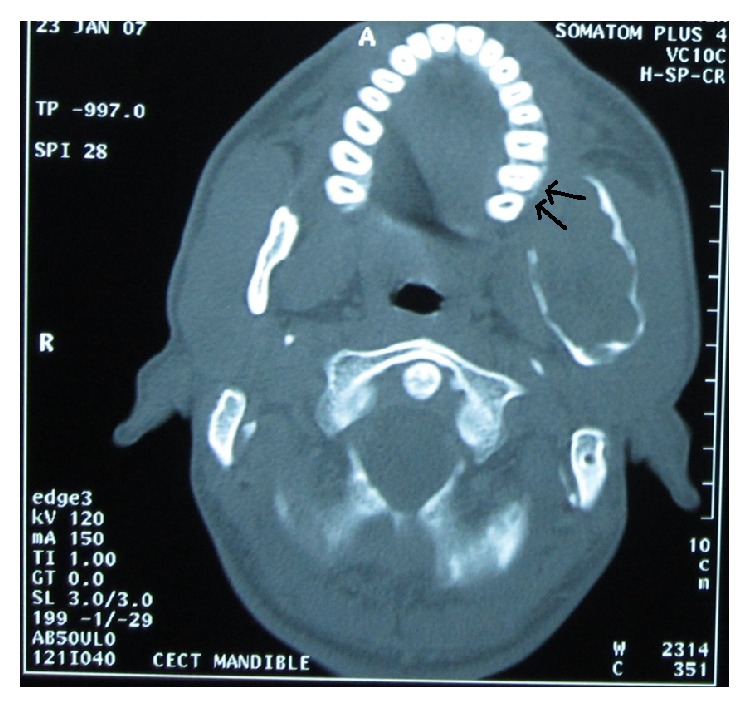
Transverse section of CT showing deflection of the left maxillary arch medially near the tuberosity.
